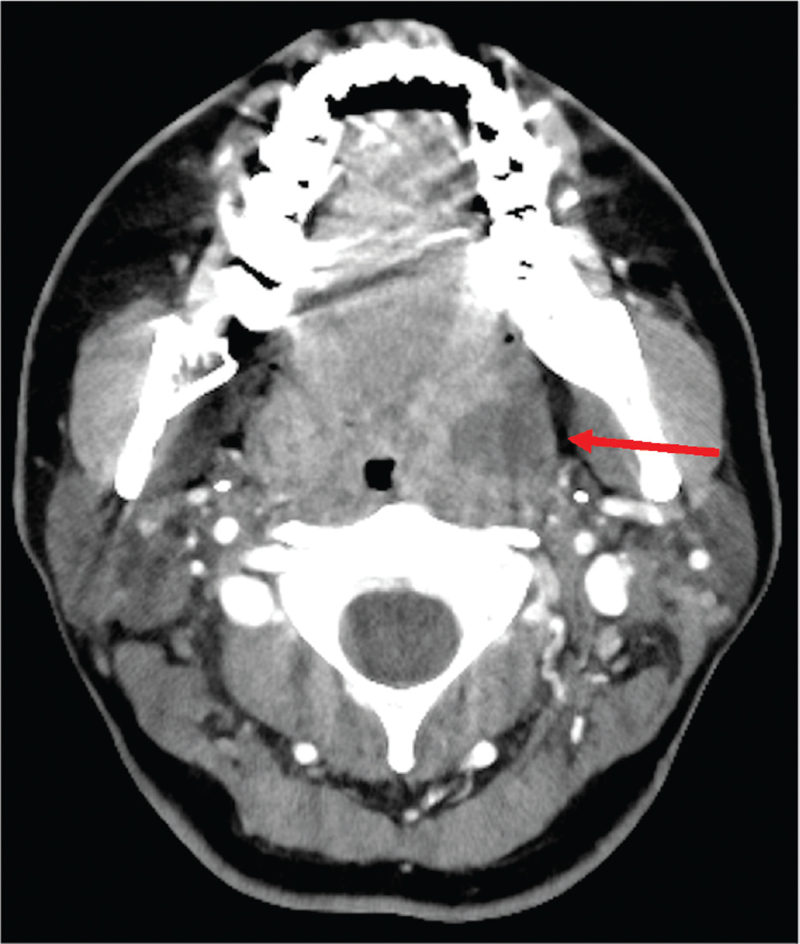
Contrast-enhanced computed tomography (CECT) in the axial view showing a low-density lesion (17.5 mm × 14.7 mm) with a ring-enhanced layer from the left-side tonsilla to the upper pharynx (red arrow).
